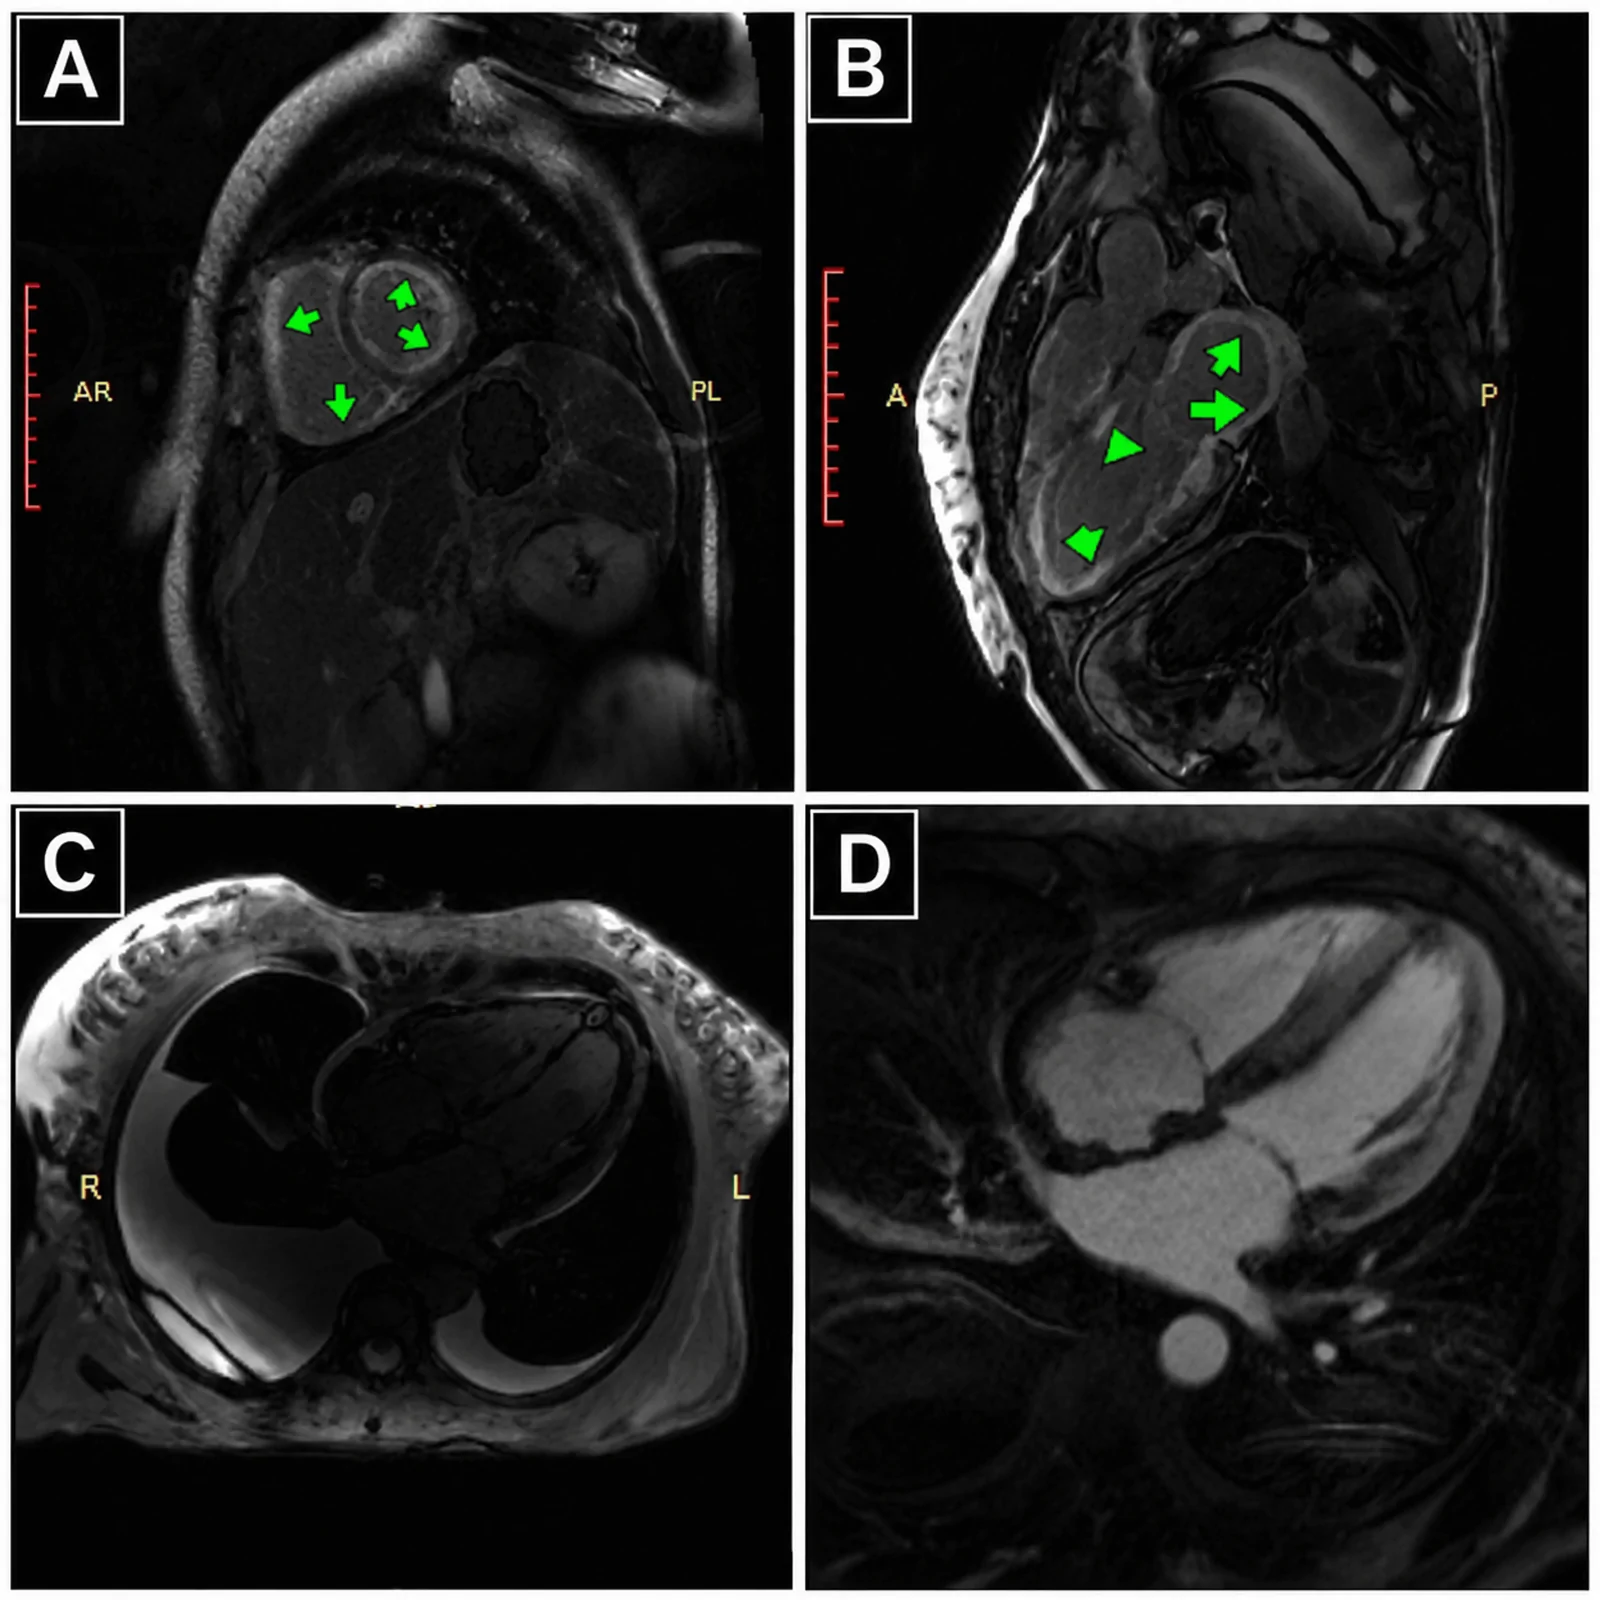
Cardiac magnetic resonance imaging. A: Short-axis view demonstrating diffuse subendocardial LGE in both ventricles (arrows). B: Three-chamber view demonstrating also diffuse subendocardial LGE in the left ventricle and left atrium. C and D: Four-chamber views demonstrating biventricular hypertrophy LGE: Late gadolinium enhancement
